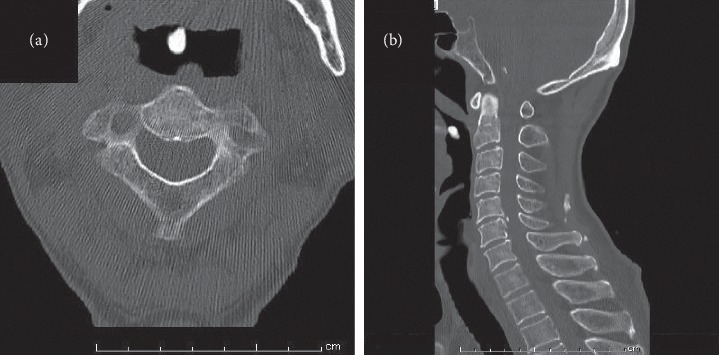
Computed tomography scan showing a well-defined, rounded, high-density mass with a tiny pedicle on the base of the tongue: (a) axial and (b) saggital.
